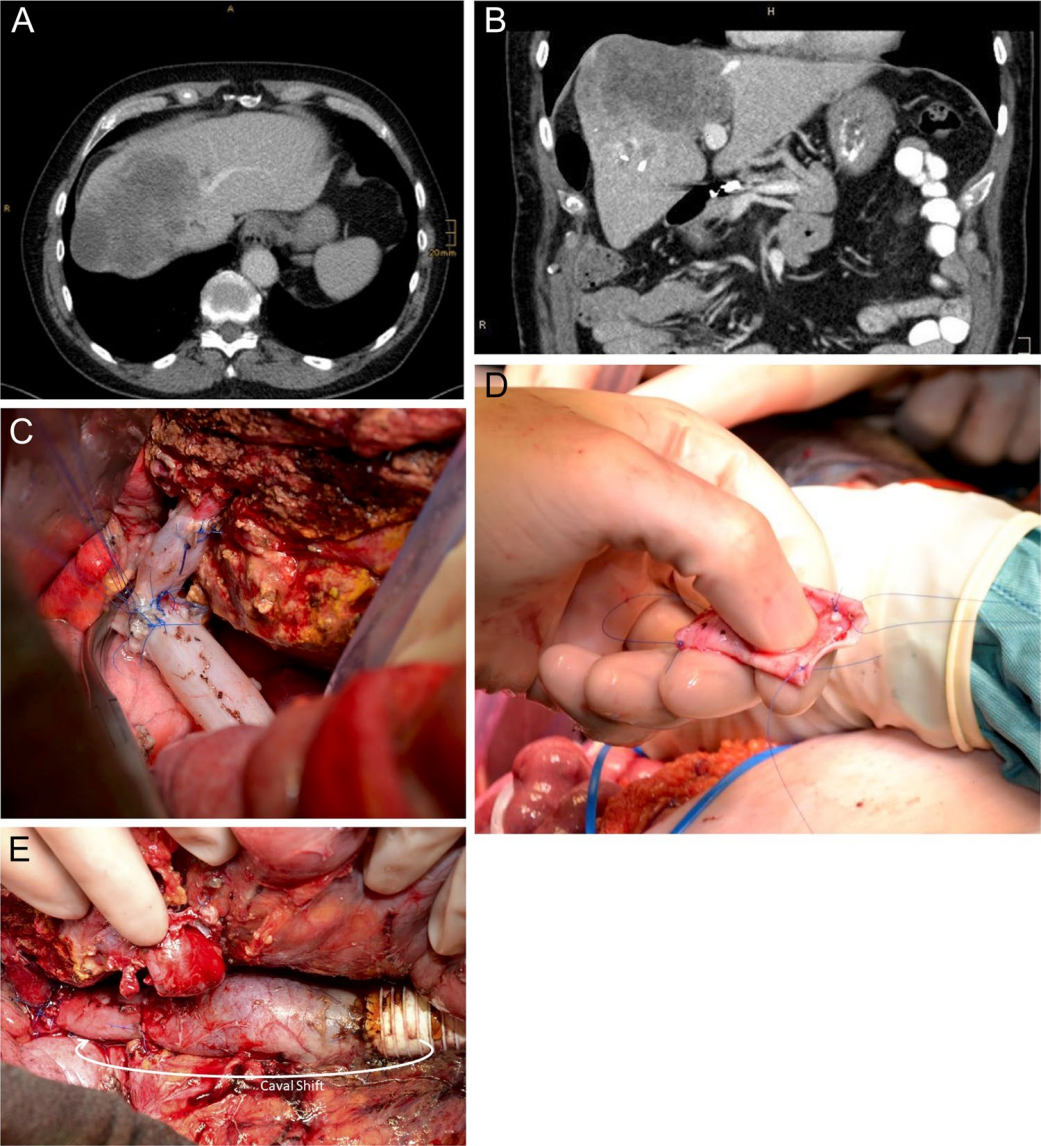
Patient with a cholangiocarcinoma infiltrating the vena cava and the right, middle, and left hepatic vein. Patient received extended right hemihepatectomy with reconstruction of the left hepatic vein by using a segment of the left internal jugular vein and reconstruction of the vena cava with a caval shift procedure as ante situm procedure. a and b Patient’s CT scan showing cholangicarcinoma infiltrating vena cava and all three hepatic veins. c Reconstruction of the vena cava after removing the tumor by extended right hemihepatectomy with insertion of an autologous caval segment. The left hepatic vein is reconstructed by interposition of left internal jugular vein. d Autologous segment of the infrarenal vena cava for reconstruction of the proximal IVC and venous confluence. e Caval shift procedure: adequate segment of infrarenal vena cava is resected and replaced by a ringed synthetic graft (e.g., GoreTex®). Picture shows the reconstruction of the infrarenal segment resection of the IVC on the right and the reconstructed IVC by the use of the autologous vena cava segment on the left
